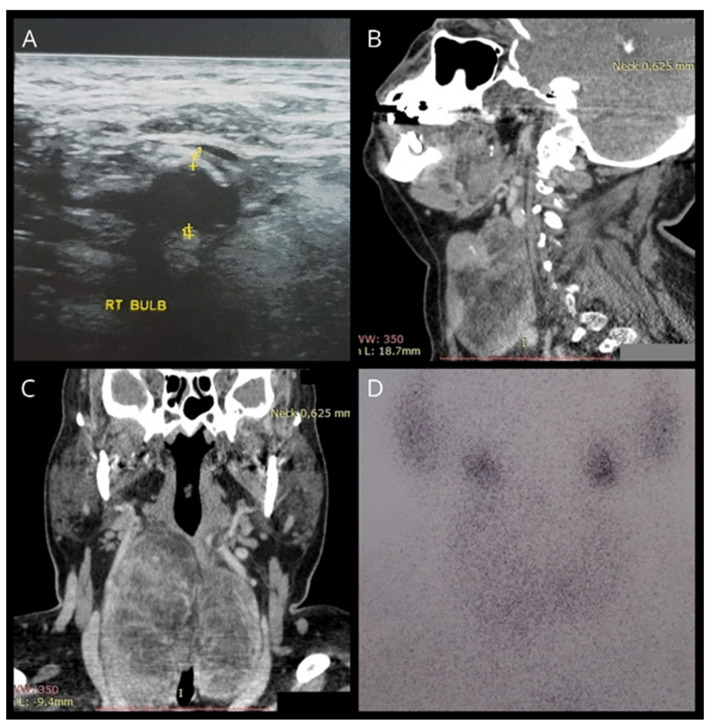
(A) Ultrasonography showing a hyperechogenic thyroid gland. (B,C) CT findings indicating a diffusely enlarged thyroid with hypodense areas and intrathoracic extensions. (D) Tc^99m^ scintigraphy showing heterogeneous uptake of the isotope with diffuse hypofixation.
